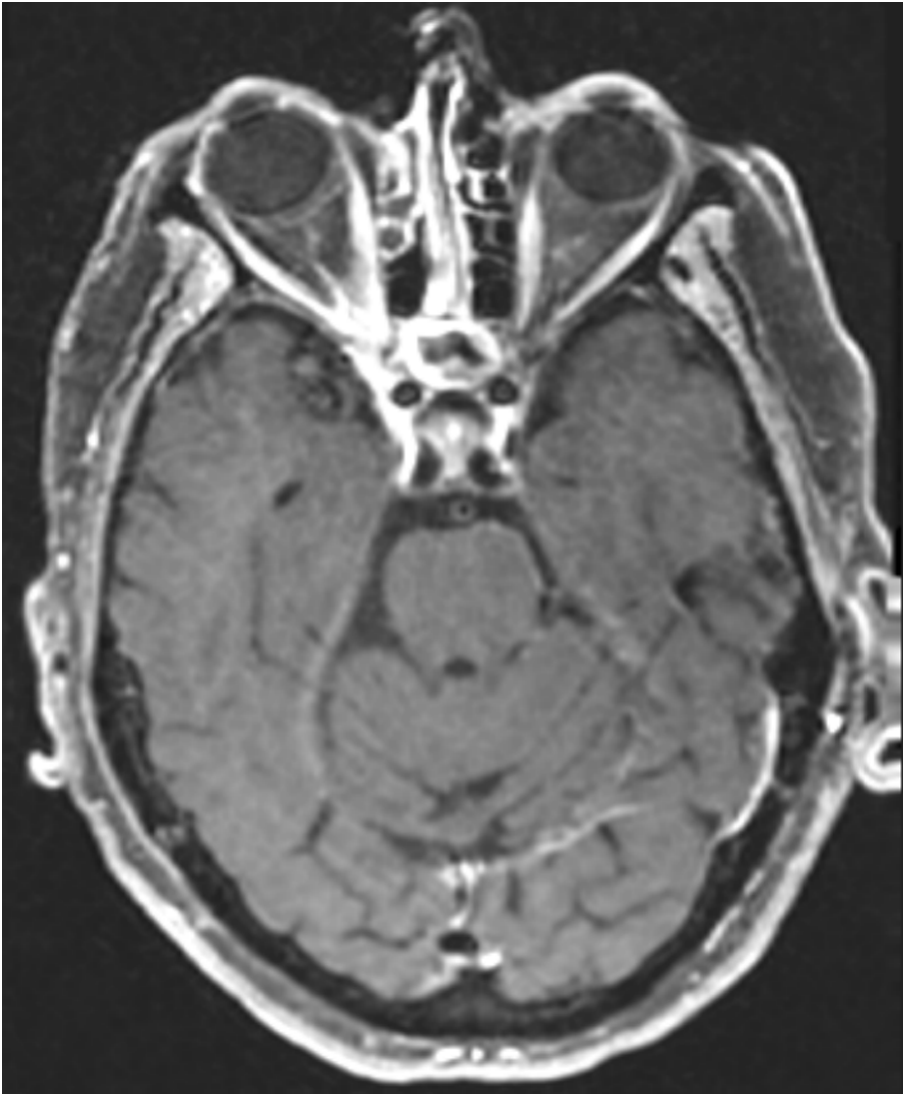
MRI of the brain, axial view postcontrast T1 fat-suppressed CUBE, demonstrating erosive defect in the right sphenoid sinus wall, allowing for phlegmonous extension of infection into right orbital apex.
